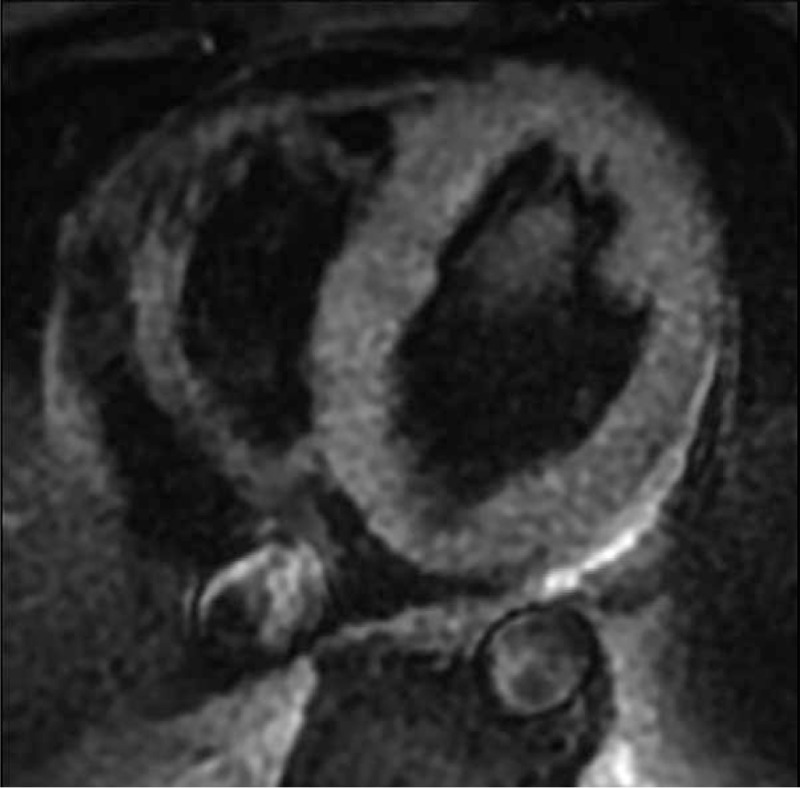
T2-weighted STIR images of transverse section showed no significant high signal (myocardial edema) of the ventricle wall.
